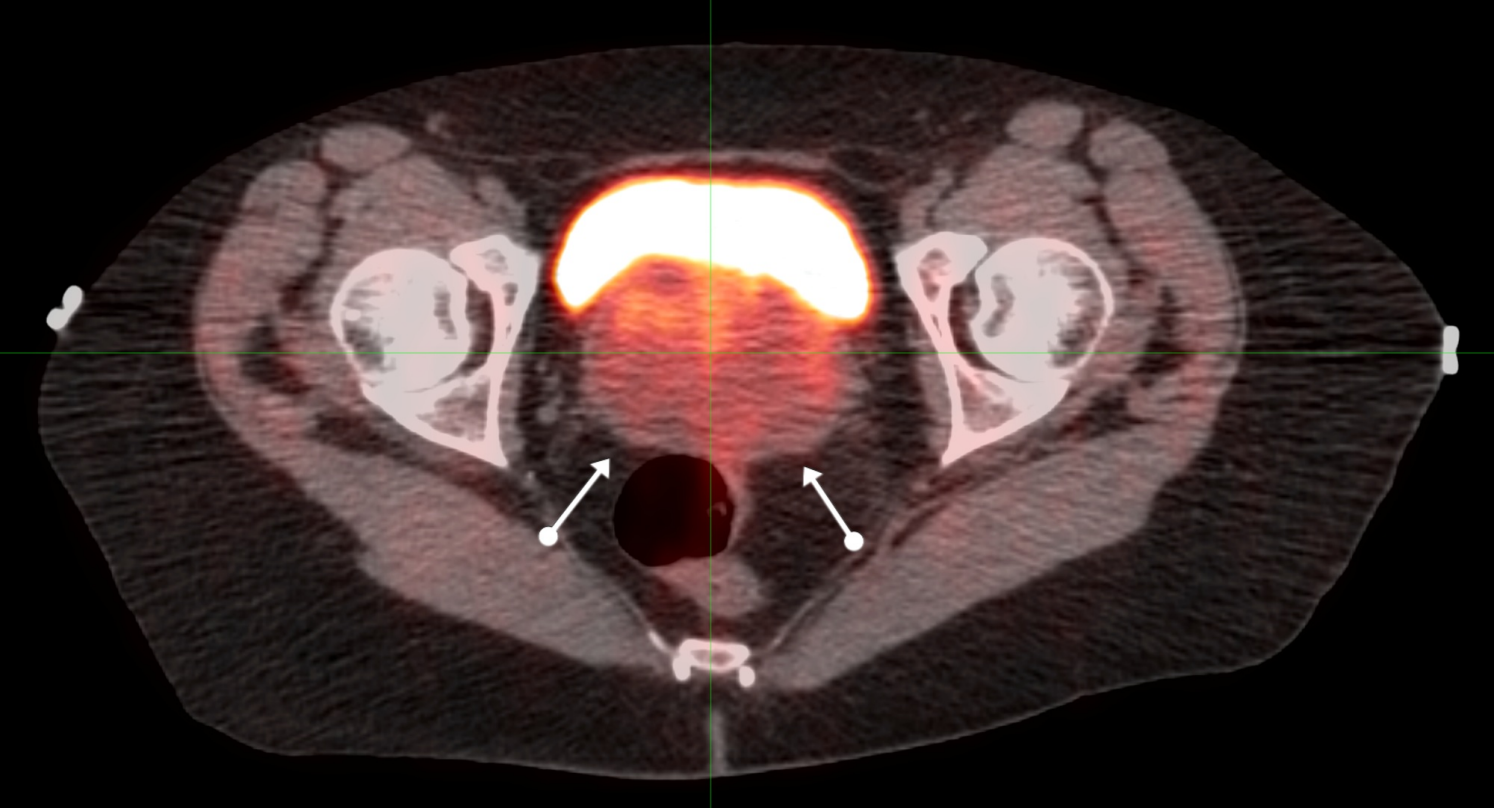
Sequential axial PET/CT images of pelvic malignancy: mid positron emission tomography-computed tomography: PET/CT
